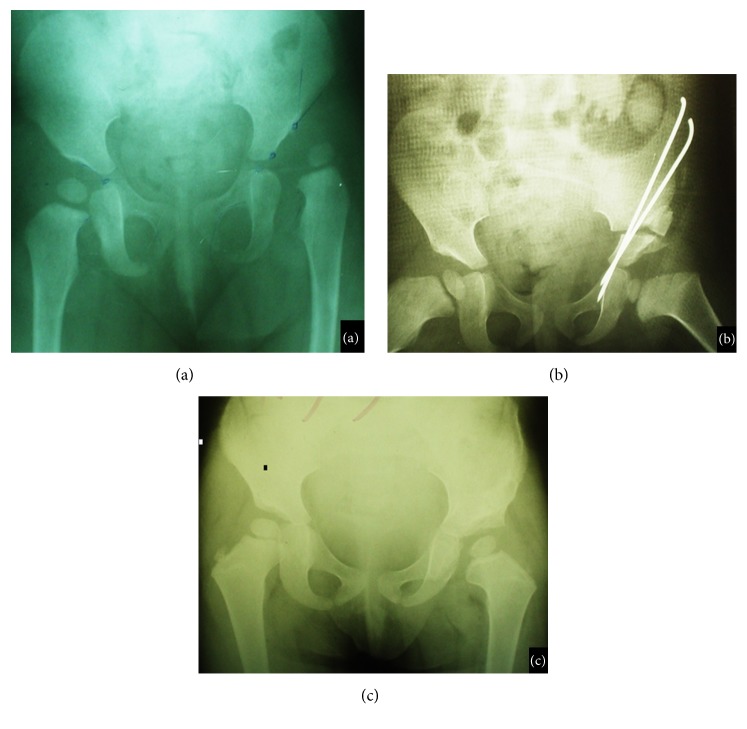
(a) Preoperative radiograph in a 23-month-old girl presetting with left developmental dysplasia of hip. (b) Immediate postoperative radiograph. (c) Radiograph taken at 3.5-year follow-up.
